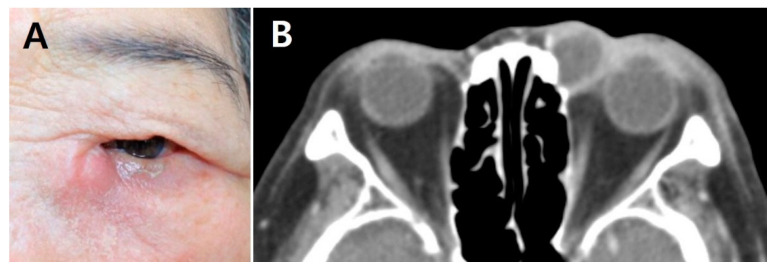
Representative facial photograph and computed tomography (CT) imaging of an AD patient. (A) External photograph of an 80-year-old woman who presented with pain, redness, and swelling around left the lacrimal sac area. (B) CT scan showing peripheral enhancing cystic lesion in the left medial canthal area with enhancing soft tissue swelling in the adjacent periorbital area.
